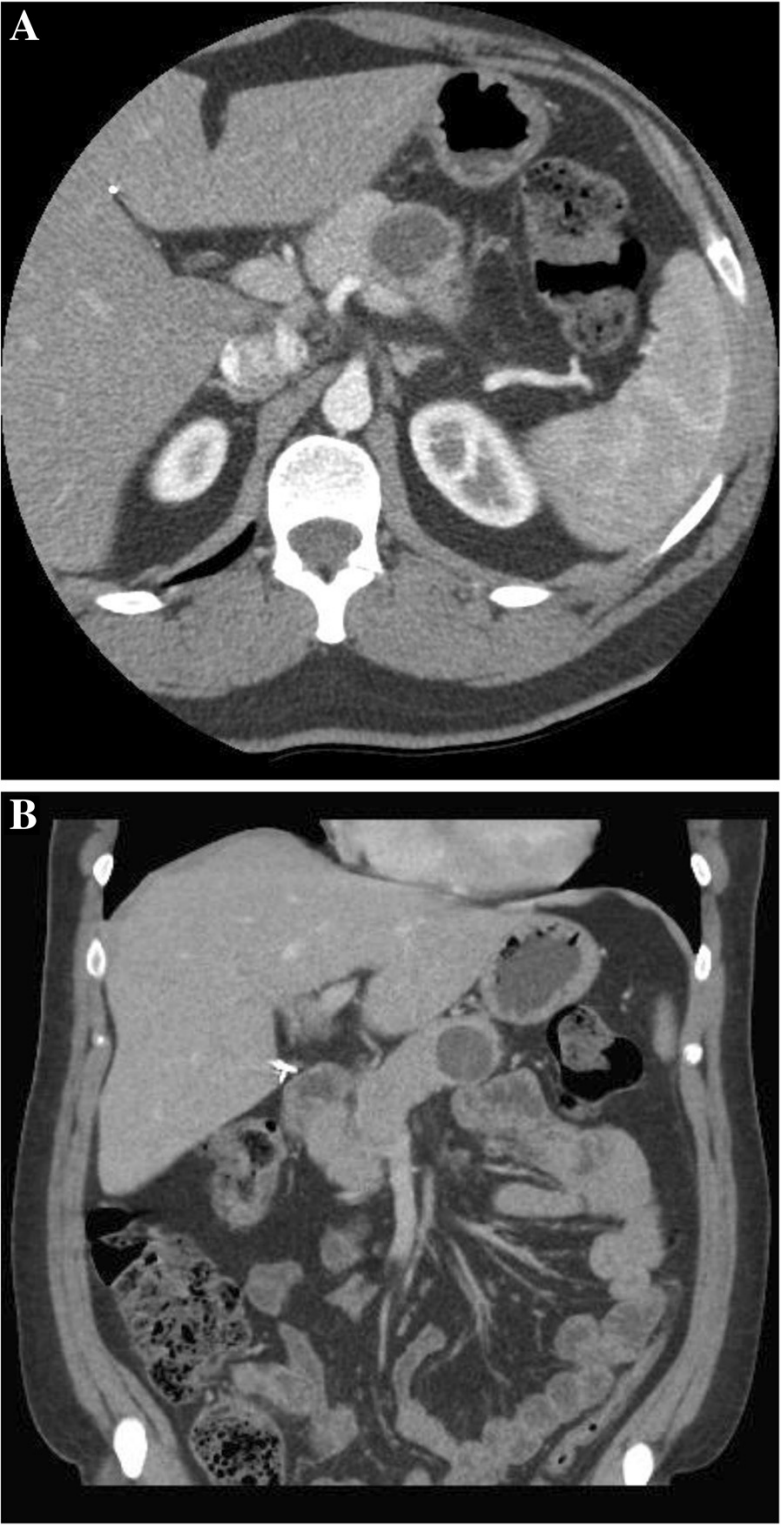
Abdominal CT with pancreatic protocol showing cystic pancreatic mass. a Arterial phase CT axial slice through the pancreatic body shows a cystic mass within the body region. b Coronal reconstruction portal venous phase shows a cystic mass confined to the pancreatic parenchyma
